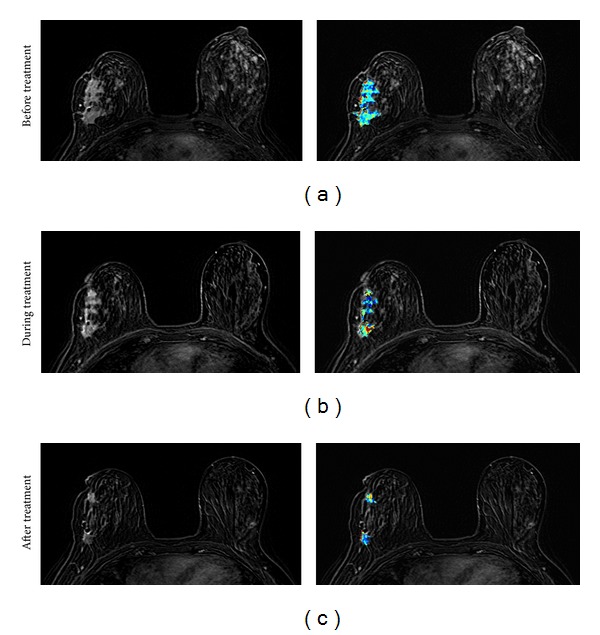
A 41-year-old patient with non-mass-like enhancement lesion (invasive ductal cancer with lobular features). From (a) to (c), the contrast-enhanced (subtraction) images selected from the same level in pretreatment, F/U-1, and F/U-2 MRI are shown. The tumor boundary cannot be clearly determined, and thus the extent of the tumor cannot be measured precisely. While the disease extent does not change much in F/U-1, it is noticeable that the area of the enhanced tissues is smaller in F/U-2 after completing NAC. The right panel shows the corresponding color-coded *K*
